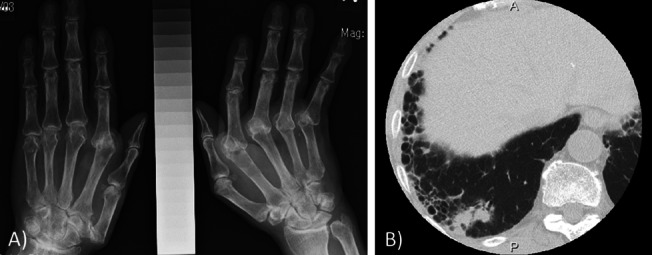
Radiological findings obtained at another hospital before admission. A X-ray of the hands obtained 13 months before admission showed joint space narrowing at the radiocarpal joint and intercarpal joints of the left hand. Subluxation at multiple sites of the metacarpophalangeal joints was also noted in both hands. B Chest CT taken 11 months before admission revealed a solitary nodule in the right lower lobe. Mild fibrosis showing subpleural honeycombing was observed in the same area
